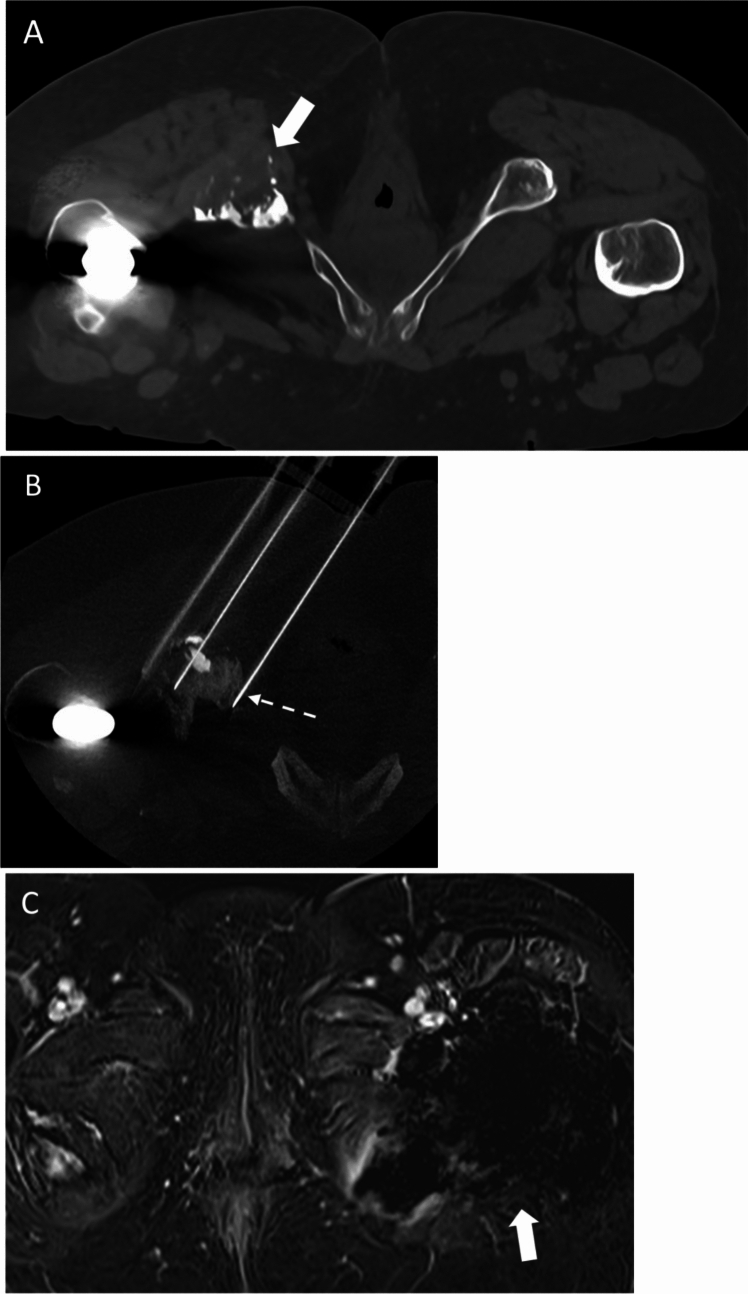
A 56-year-old man with advanced RCC and painful right ischial bone metastasis. a Unenhanced axial CT pelvis showed aggressive lytic bone metastasis at right ischium. b Under CT guidance, electrochemotherapy, i.e. reversible electroporation with intravenous bleomycin administration, was used to treat the right ischial bone metastasis. Electroporation needle was indicated by *dashed white arrow*. c Axial T1-weighed post-contrast MR pelvis showed necrosis of right ischial metastasis. The patient remained opioid-free for 6 months
